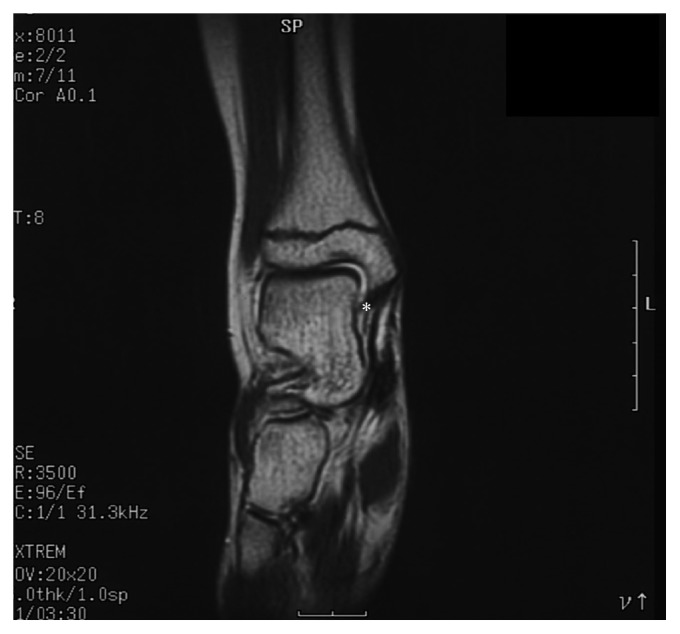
Magnetic resonance (T2-weighted) imaging of the right ankle in the patient at the age of 11 years. A small amount of fluid collection in the joint space (∗) was observed; however, there was no evidence of synovitis or destructive changes in the bone.
